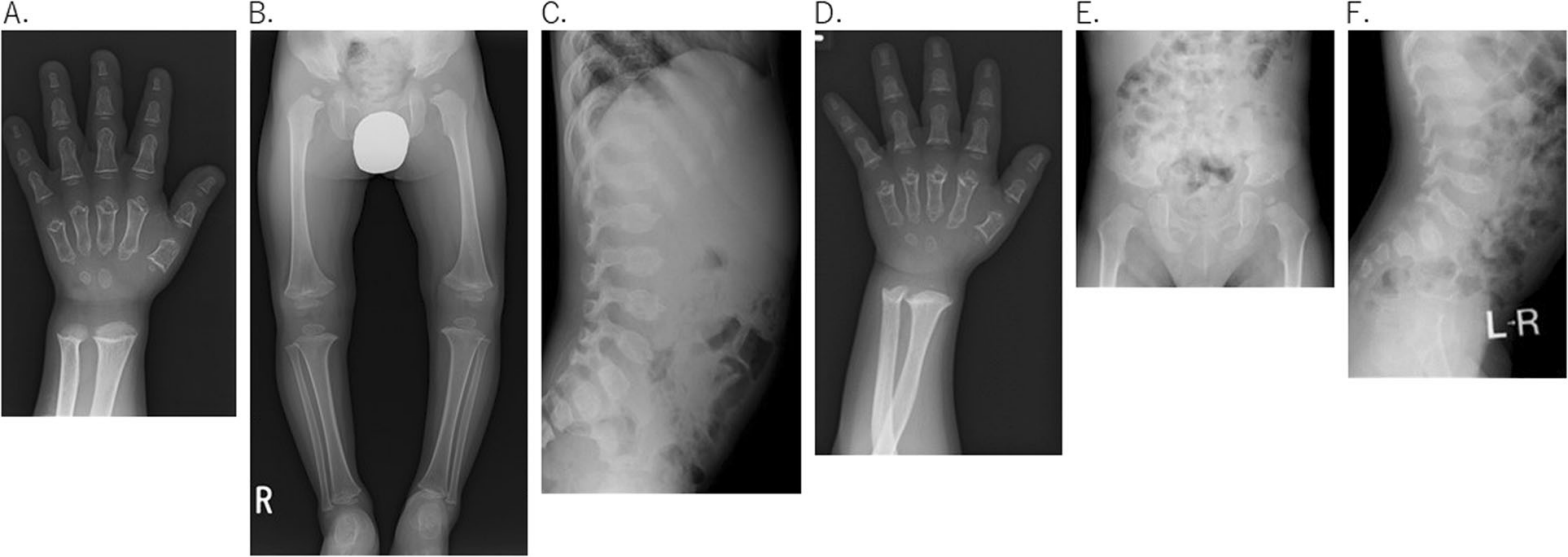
Radiographic findings of two boys with PSACH. a, b, and c show X-ray images in case 1, and d, e, and f show X-ray images in case 2
